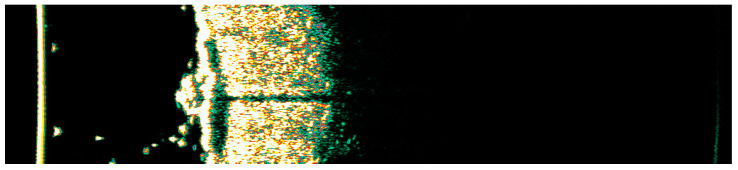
Ultrasonographic image of BCC on the lower back.
